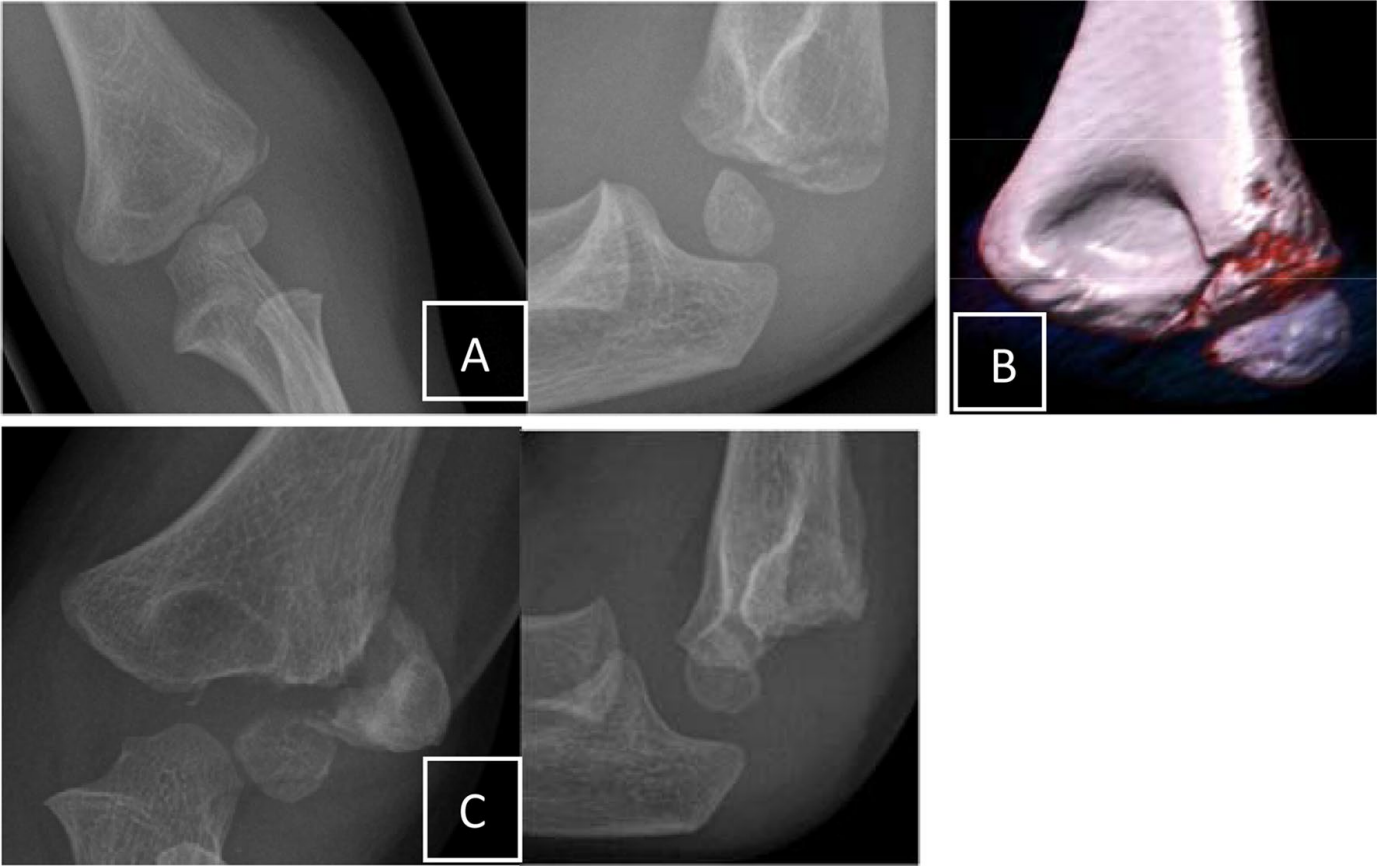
a Typical undisplaced condyle fracture in a small child. b The fracture characteristics were additionally, but unnecessarily documented with CT. No control at day 5–7 but 4 weeks later with the secondarily displaced fragment. Waiting for whatever. c Definite non-union 1 year later
